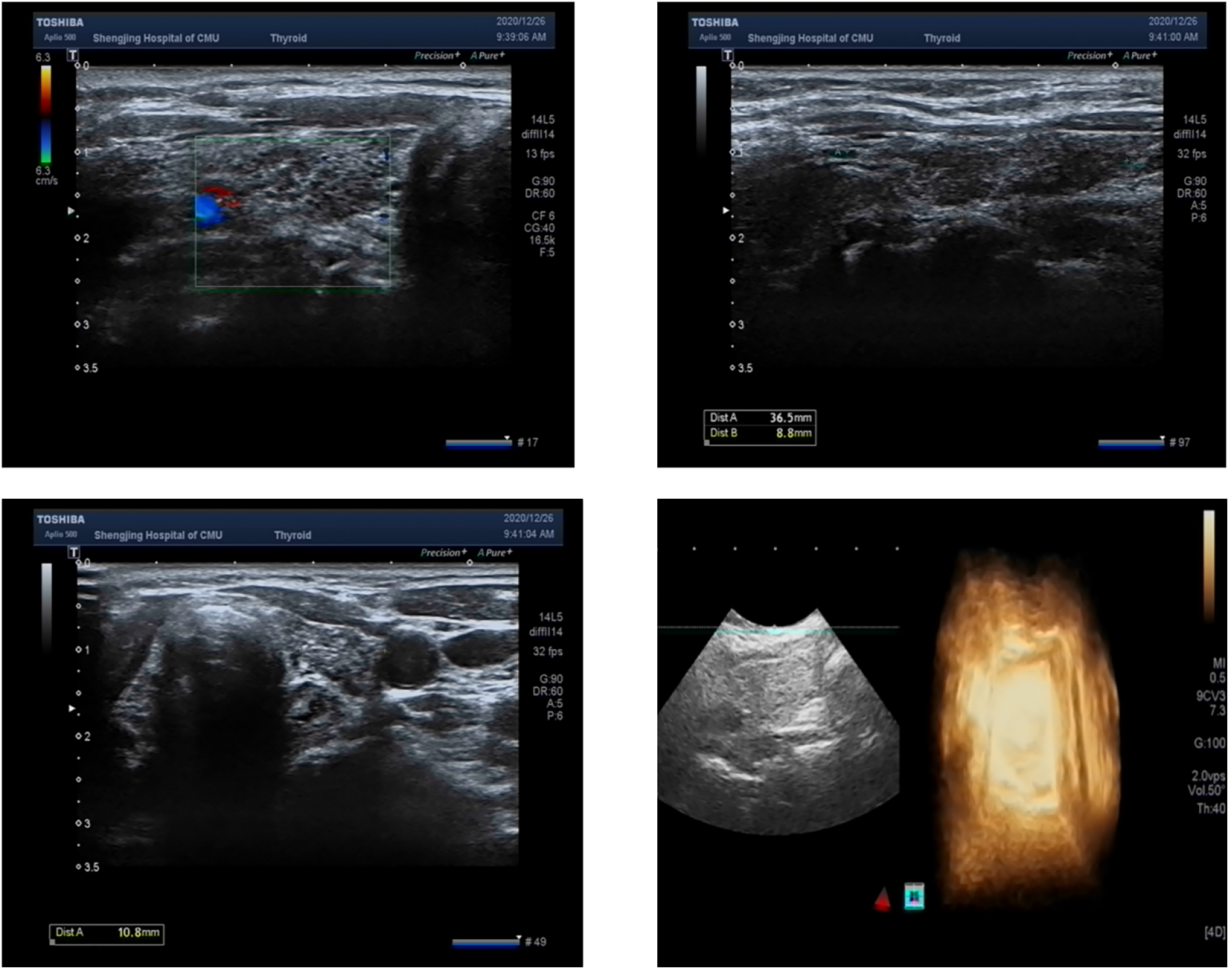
Ultrasound showing normal thyroid and parathyroid glands.
